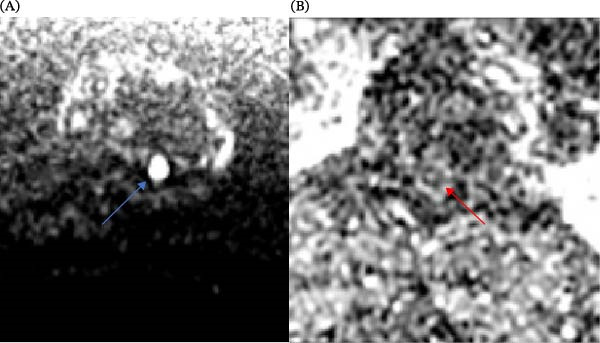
DWI (A) and ADC (B) imaging findings.
